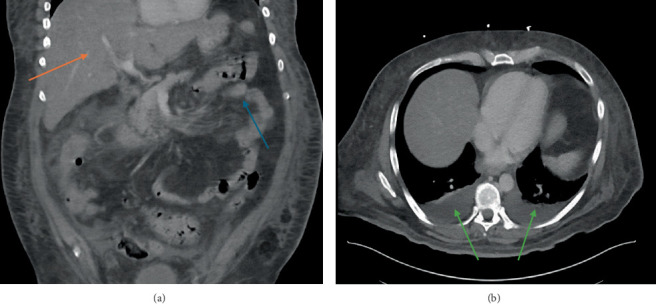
(a, b) CT scan showing splenic flexure colonic wall edema (blue arrow) along with fatty infiltration of the liver (red arrow). Bilateral pleural effusions (green arrows).
